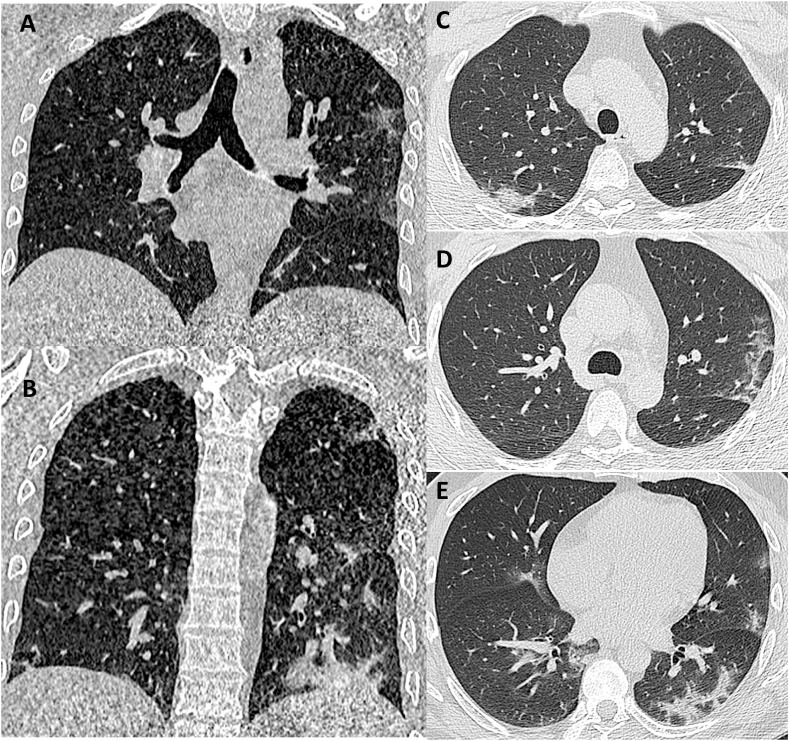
Noncontrast chest CT images of a 43-year-old man. Coronal reformatted (A and B), and axial (C–E) images show bilateral ground glass opacities in multiple lung segments and a CT-SS of 12.
